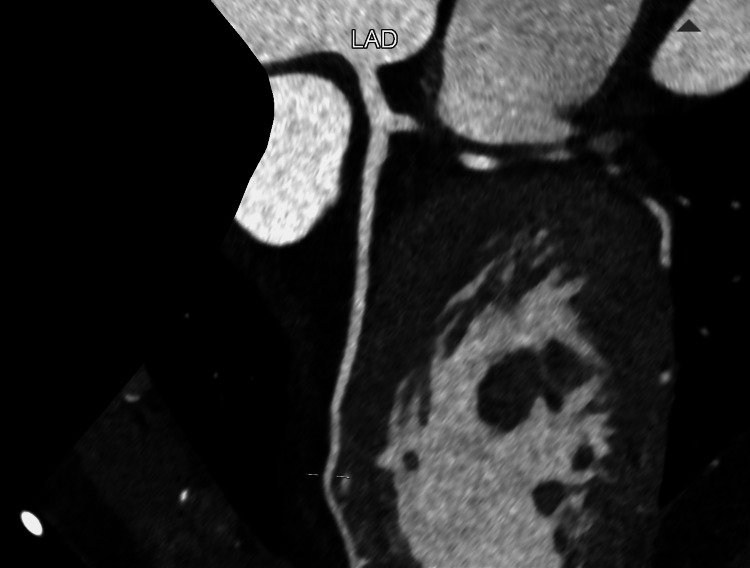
CT coronary angiogram (CTCA) showing left anterior descending coronary artery. CTCA showing the lumen is well-opacified with contrast with no visible narrowing or calcified plaques.
